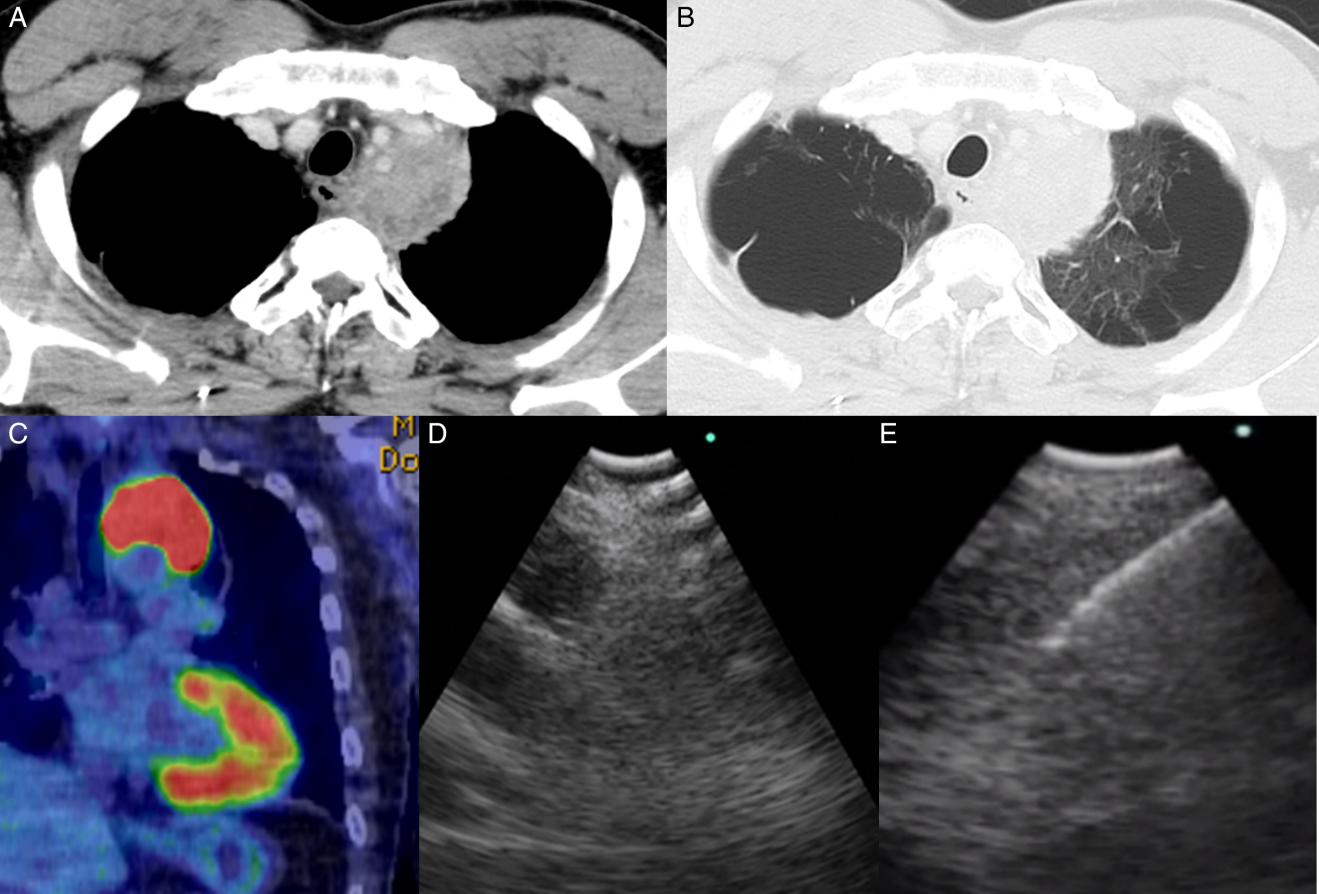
(A) Computed tomography revealed a superior mediastinal mass 60 mm in the longest diameter. (B) Emphysema was evident on computed tomography. (C) Fluorodeoxyglucose‐positron emission computed tomography revealed a hypermetabolic lesion at the location of the superior mediastinal mass. (D) The inadequate endobronchial image of the superior mediastinal mass. (E) The endo‐oesophageal image of the puncture procedure showing the needle within the superior mediastinal mass.
